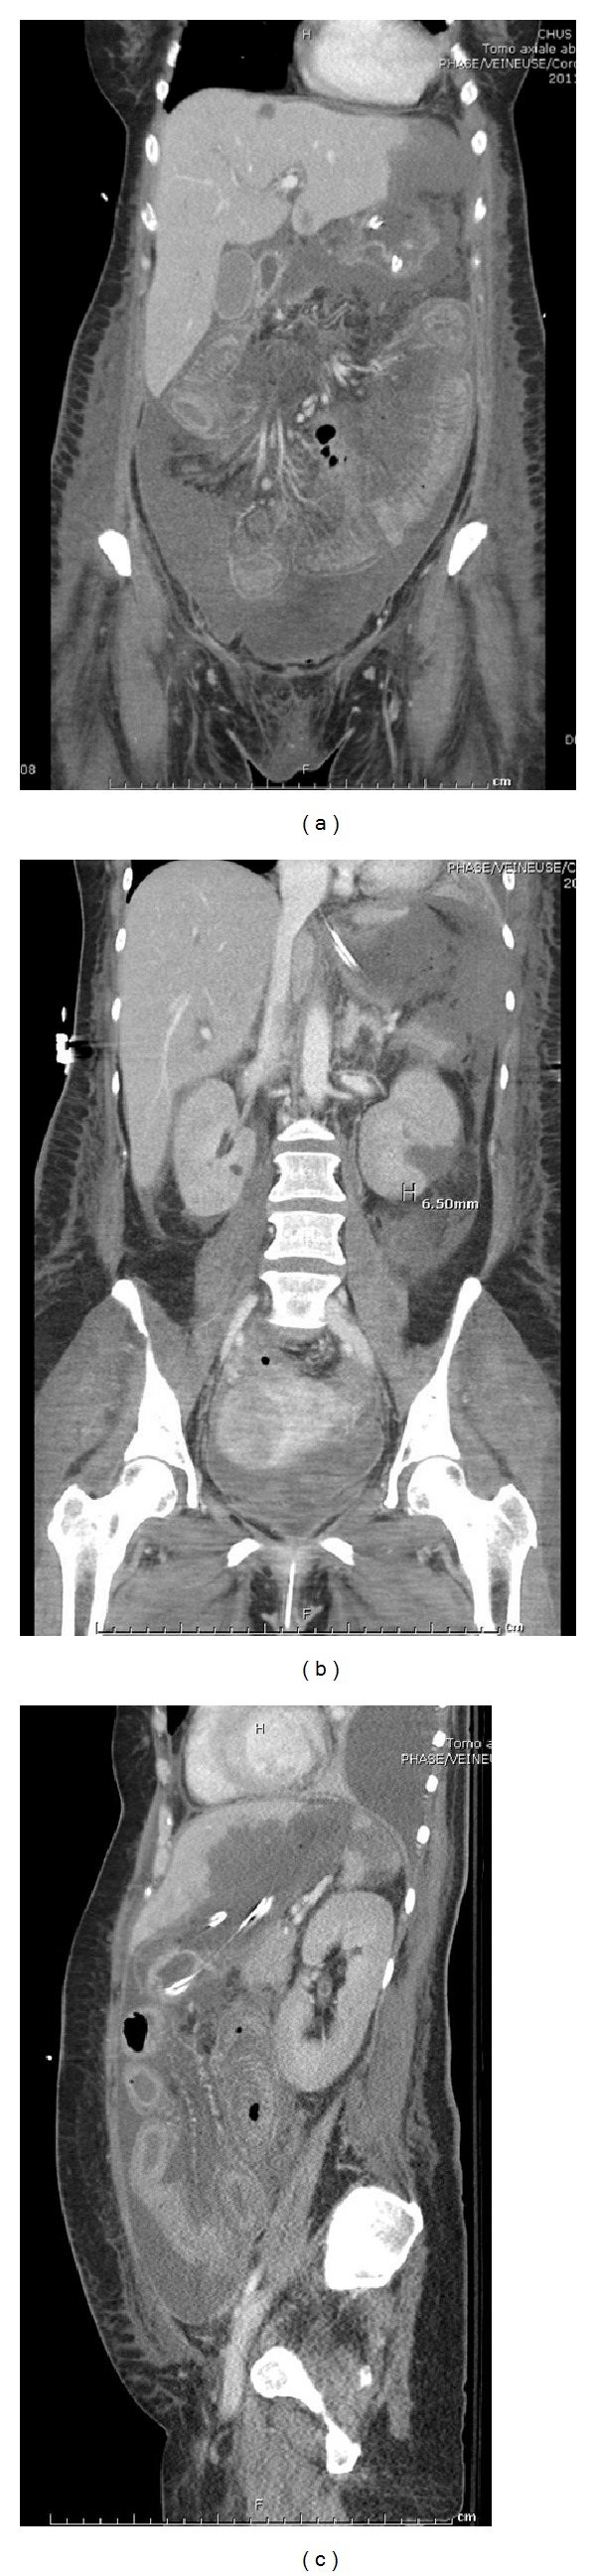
An abdominal CT scan documented a lack of enhancement of the distal portion of the small bowel, including the ileostomy, compatible with ischemia (a). Numerous splenic, hepatic, and renal infarcts were visible (b and c).
